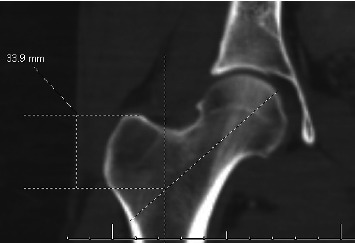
Measurement of GT height via CT image in EMR/PACS, with 33.9 mm representing the height of the GT.
